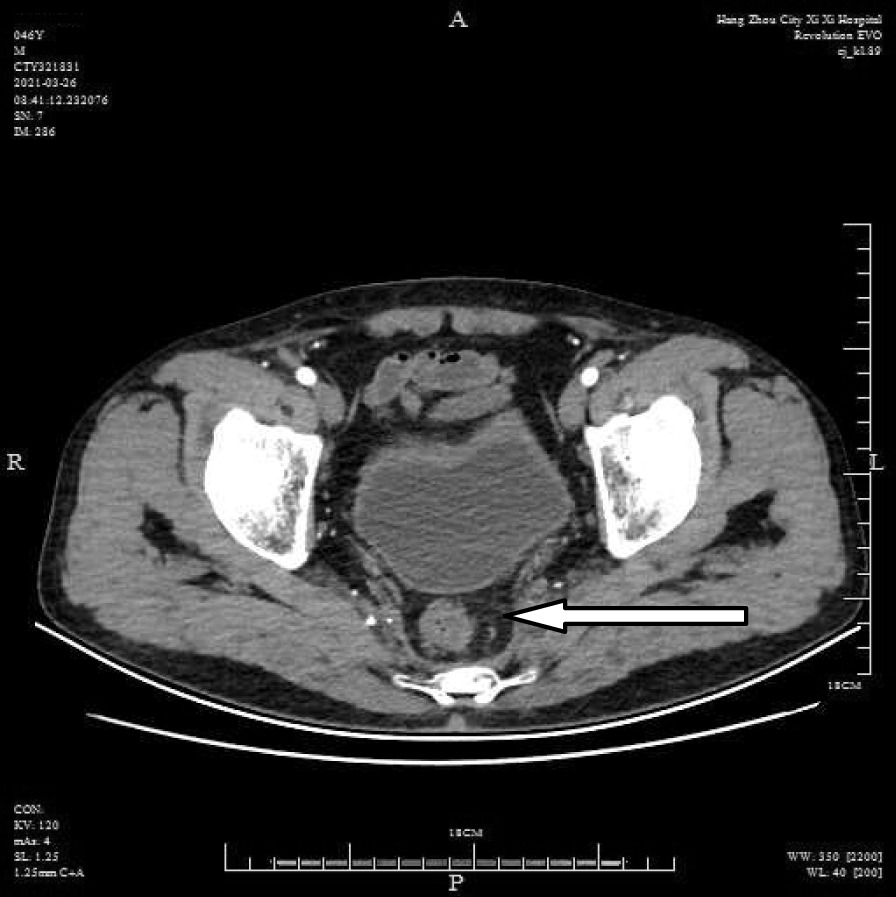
Retroperitoneum normal in CT after one month treatment
